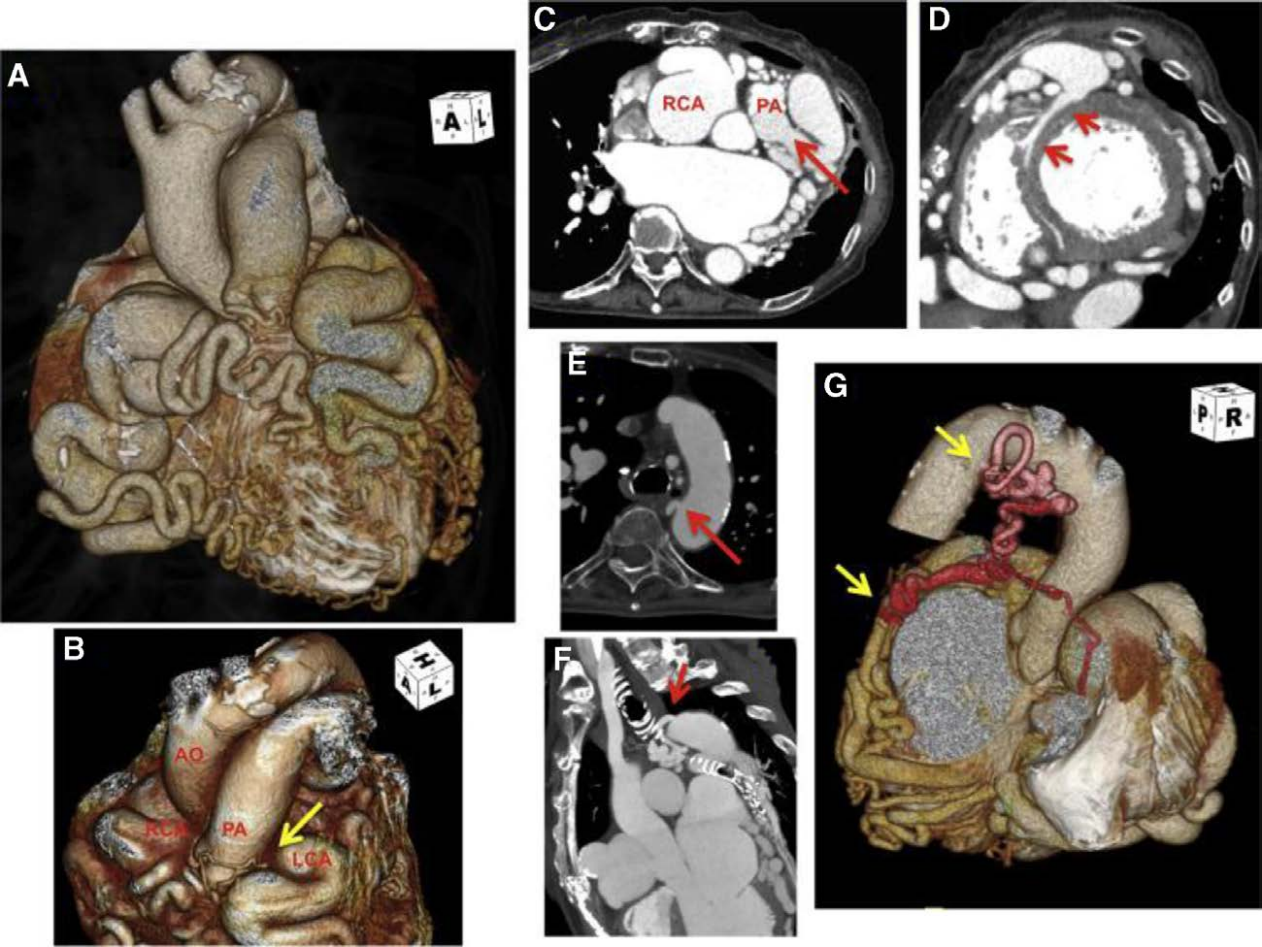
Volume-rendering (VR) images (A, B) and slice views (C) of coronary computed tomography angiography are shown. (A, B, C)Coronary MDCT showed anomalous origin of LCA from the PA (arrow), where RCA and LCA are tortuous and dilated; (C) tumor-like dilated RCA emits multiple collateral vessels (arrow), (D) Short axis slices showed markedly dilated septal branch perforating through myocardium; (E, F)the bronchial artery forms collateral branches from the aortic arch (arrow) to the left atrium and (G) supplies the left circumflex artery (arrow).^[5]^ LCA = left coronary artery, MDCT = multi-detector computed tomography, PA = pulmonary artery, RCA = right coronary artery.
